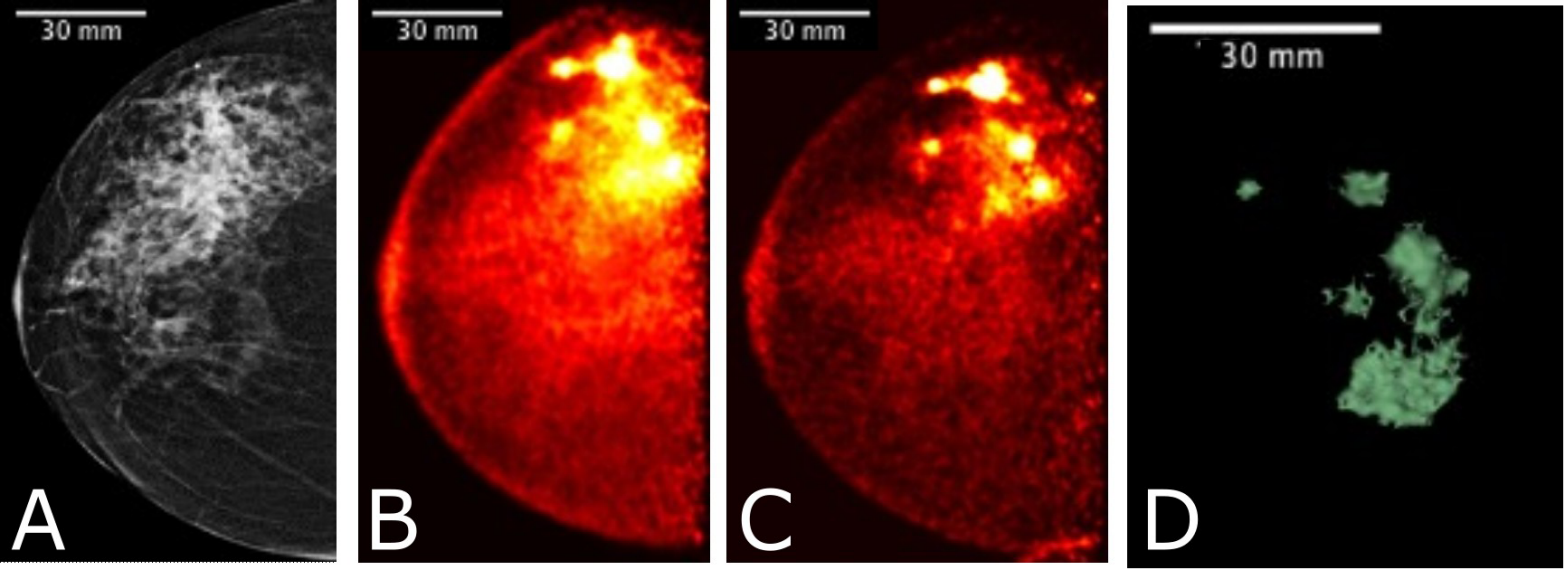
Images acquired for a 61 year-old female with right breast multifocal invasive and *in situ* ductal carcinoma. Images show the same breast in: (A) FFDM in the CC plane with extensive distortion; (B) 3-D Radialis PET image in the CC plane 1 hour after 178 MBq ^18^F-FDG injection; (C) 3-D Radialis PET image in the CC plane where multiple distinct regions of contrast uptake are still evident 4 hours after ^18^F-FDG injection. Mean lesion SUV corrected for lean body mass (SUV_mean, LBM_) is 1.8, with SUV_max, LBM_ equal to 3.4.; (D) image of a 3-D volume of different foci generated from Radialis PET in the CC view based upon tissue metabolism across all image slices (12).
